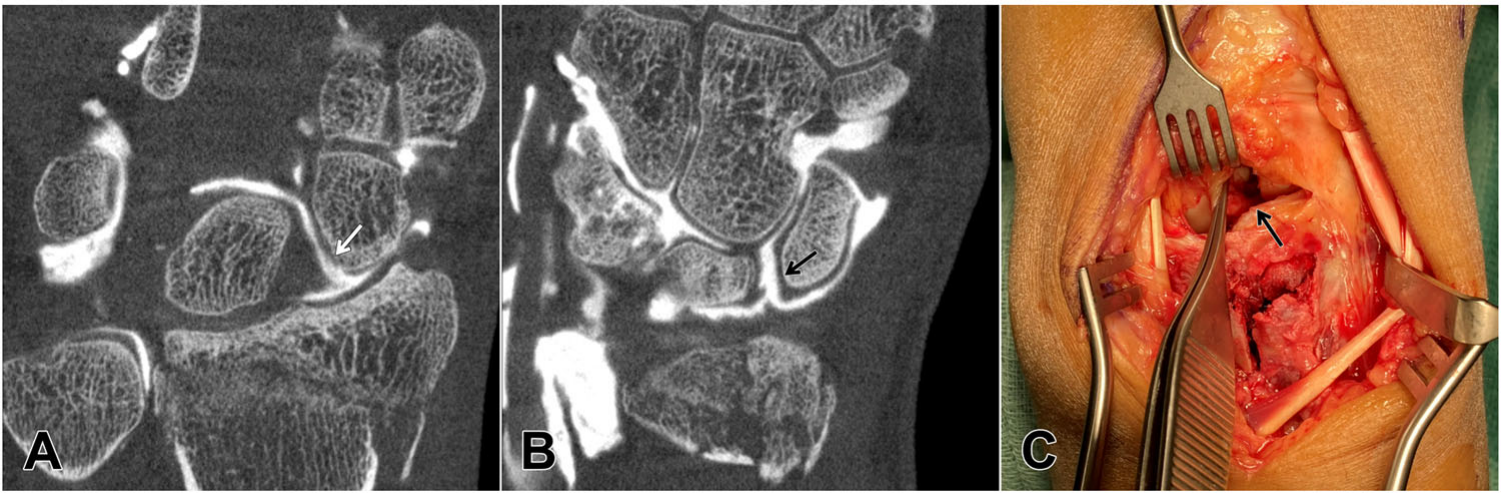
During ice skating, a 54-year-old goldsmith fell on his outstretched arm and suffered a distal radius fracture with a multi-fragmentary pattern that involved both the radiocarpal and distal radioulnar joint surface. Due to the bi-articular fracture morphology, cone-beam CT arthrography was requested preoperatively to assess the ligamentous integrity of the proximal carpal row. The minimally invasive examination displayed both the complicated fracture pattern as well as tears of the palmar (A; *white arrow*) and dorsal scapholunate ligament (B; *black arrow*). Preoperative knowledge of the destabilizing ligament rupture resulted in a dorsal approach for fracture reduction and plate fixation that allowed for simultaneous refixation of the torn scapholunate ligament (C; *black arrow*)
